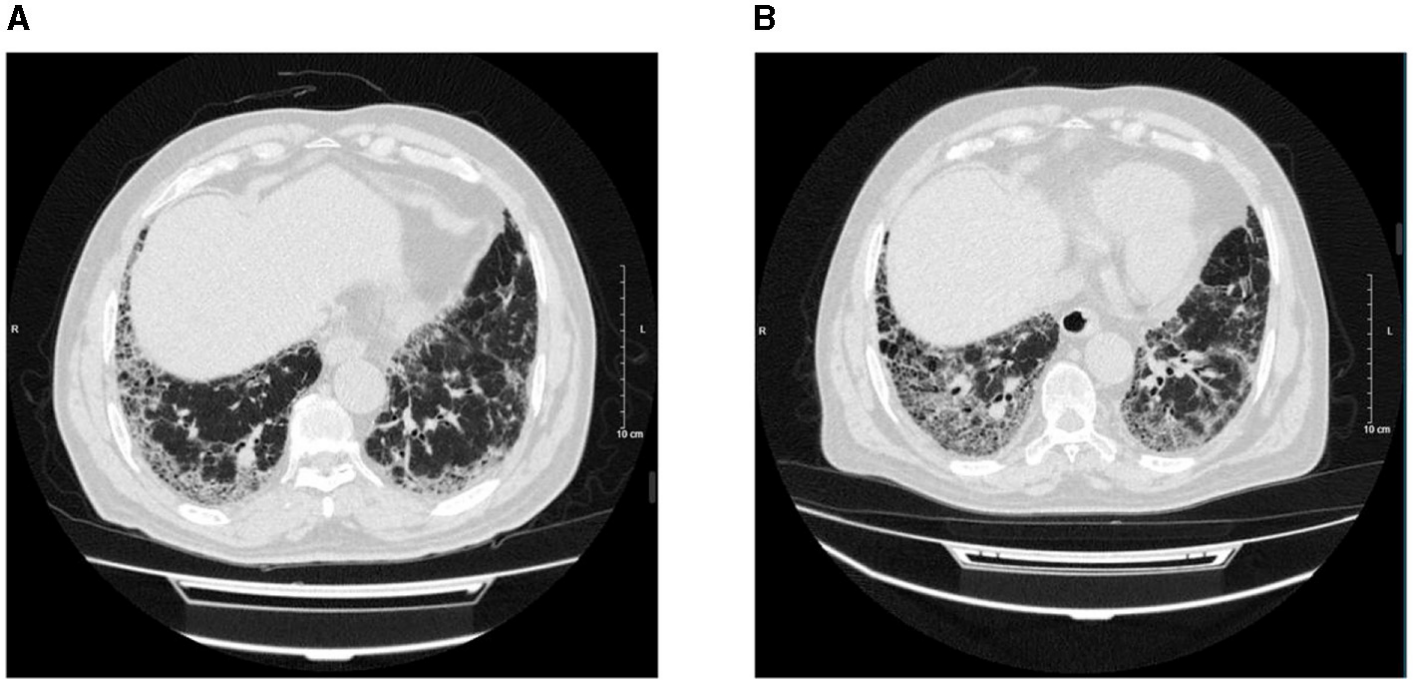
Axial HRCT images of a 75-year-old male, on 29 October 2021 (A) and 4 October 2022 (B) showed peripheral and basilar predominant progressive pulmonary fibrosis with the increased extent of reticulation, traction bronchiectasis, and honeycombing.
